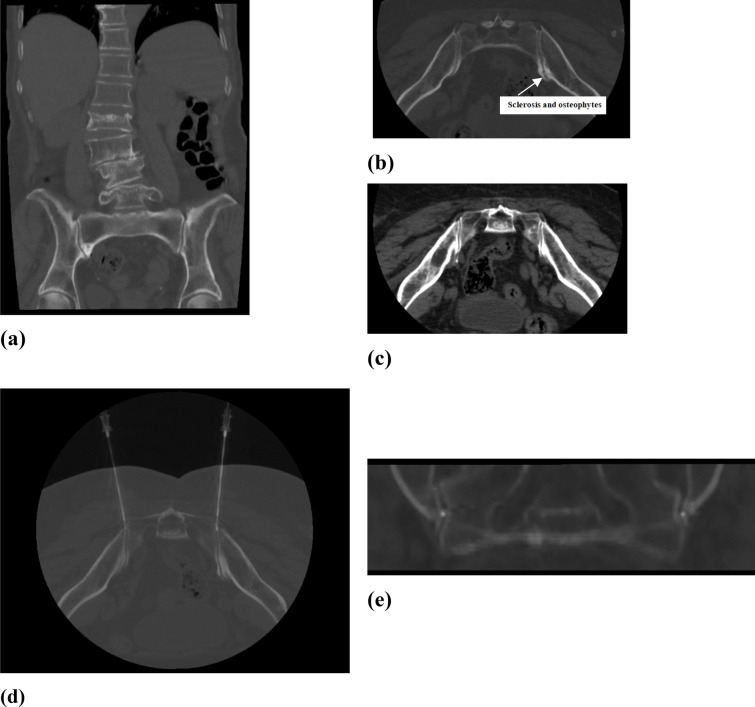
CT-guided SIJ injection in a 56-year-old female with lumbar scoliosis in the coronal plane (a), accompanied by secondary bilateral degenerative sacroiliitis, more common on the right side, with sclerosis and osteophytes, and no capsule distension seen in axial images (b and c). The procedure involved successful needle targeting (d). Image quality in (e) is lower due to the use of a low-dose acquisition technique to reduce radiation exposure
